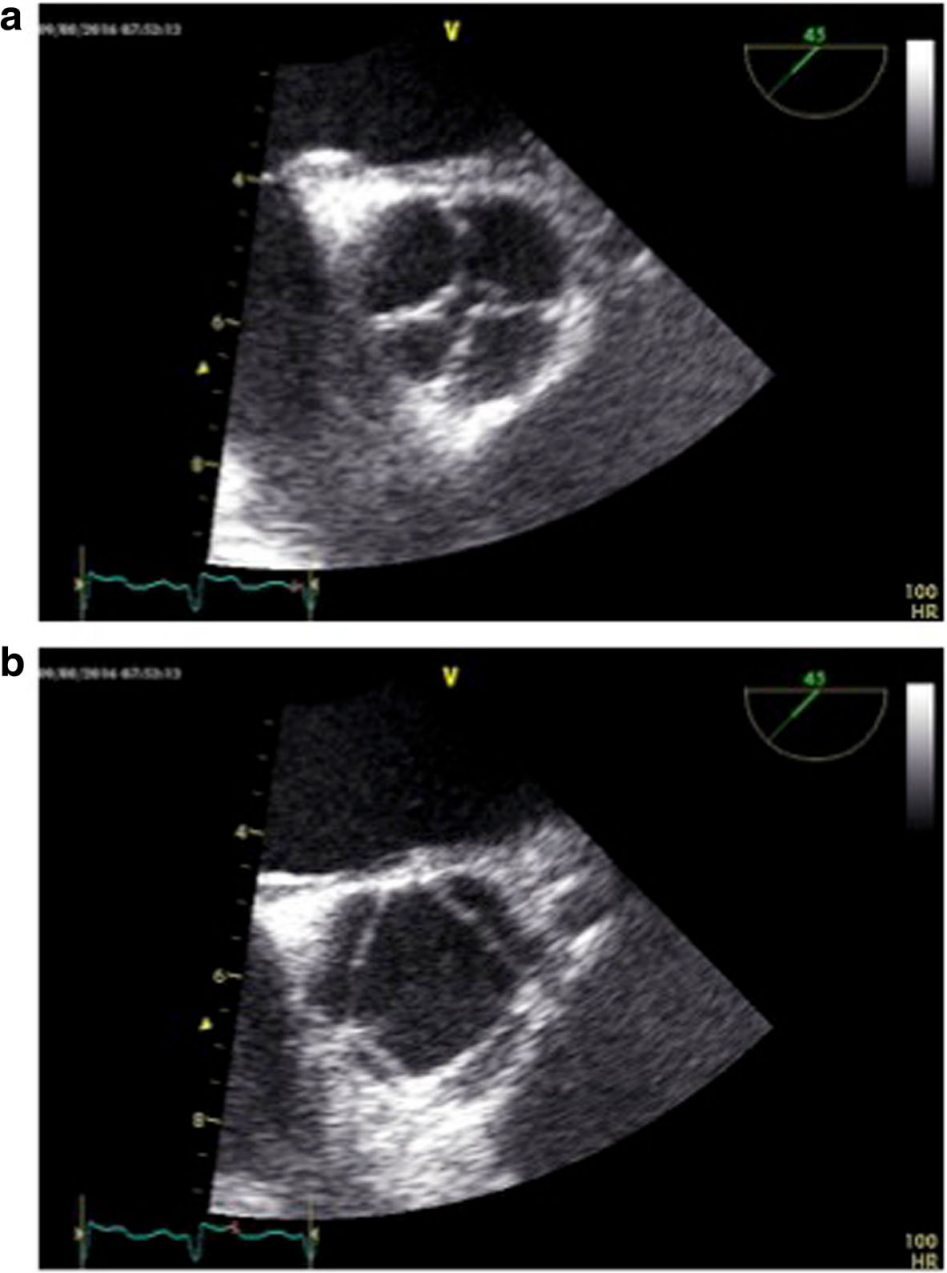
Transesophageal echocardiography: four cusps with “X” shape (a) Diastole (b) Systole
